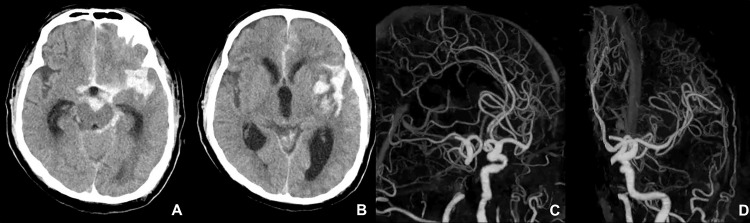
CT and CT angiography findings six hours after thrombectomy (A-B) CT reveals SAH extending from the interhemispheric fissure to the left Sylvian fissure, subcortical hemorrhage in the left insular cortex, and infarction in the left MCA territory. (C-D) CT angiography did not show any vascular abnormalities indicative of the bleeding source (C: right anterior oblique view; D: left anterior oblique view). CT, Computed tomography; SAH, Subarachnoid hemorrhage
